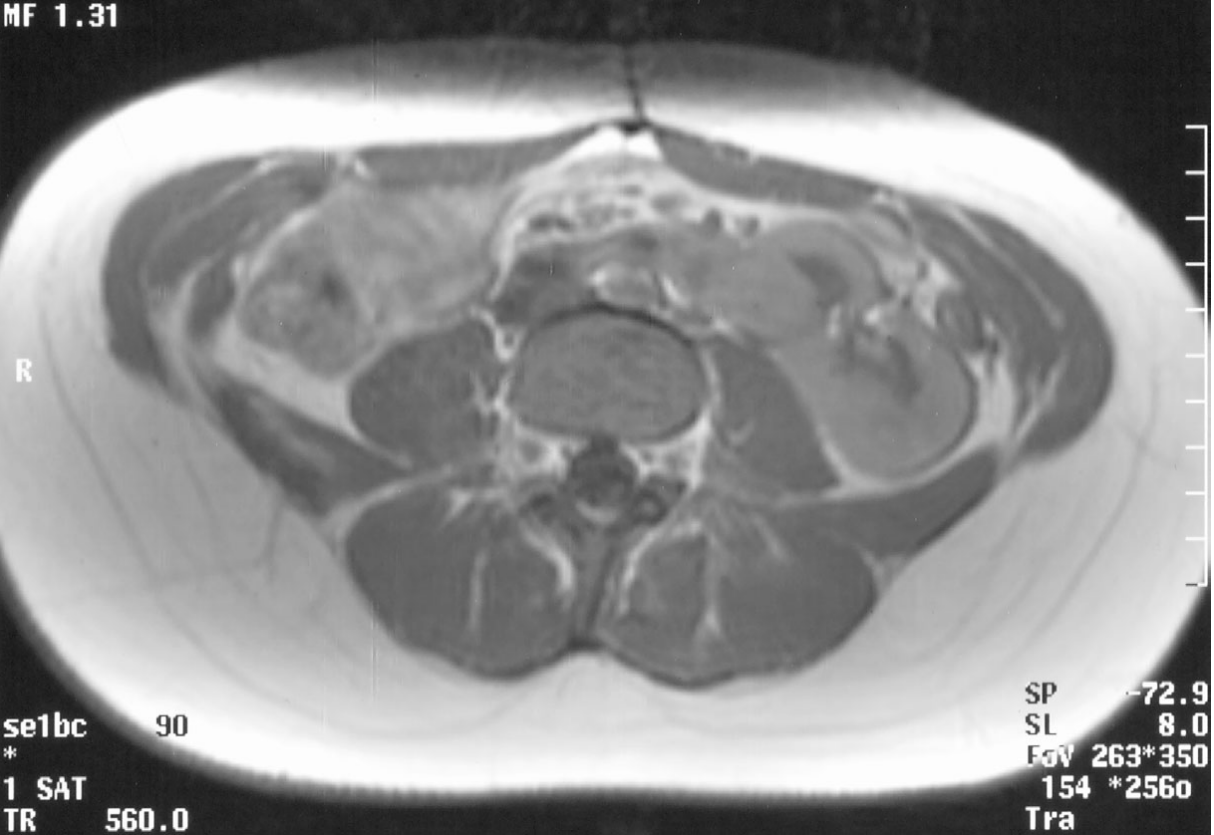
MRI Scan showing a crossed fused kidney on the left side.
